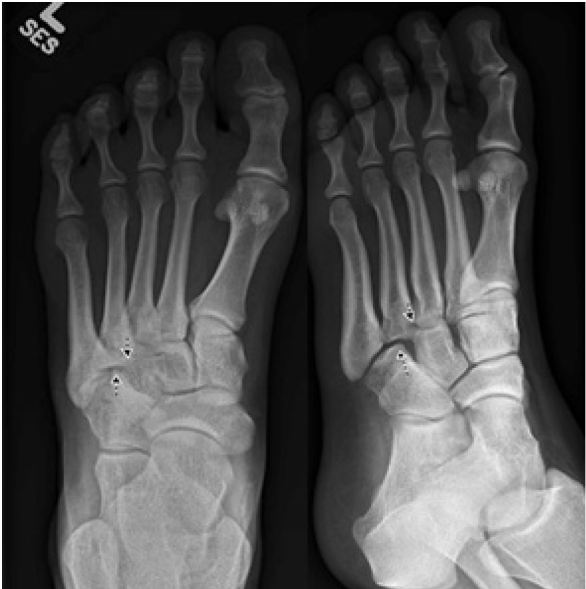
Anterior-posterior (left) and oblique (right) radiograph of the left foot with lateral displacement of the cuboid relative to the base of the fourth metatarsal (arrows).
